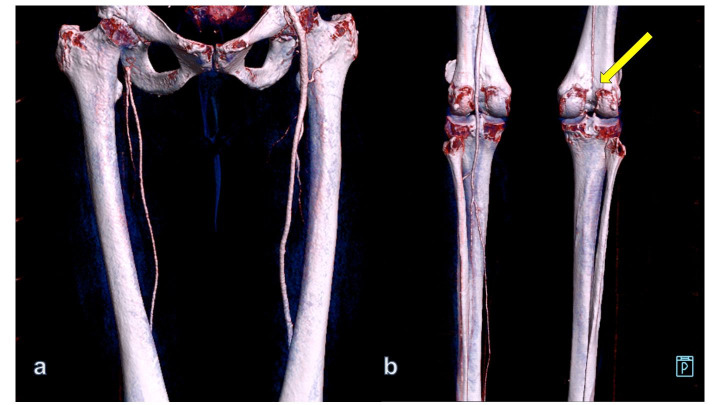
Angio CT with volume rendering 3D reconstruction showing infrafilling of a patent right superficial femoral artery (a). Total occlusion of the right popliteal artery (arrow) with absence of tibial vessels (posterior view). In the left lower extremity, all infrapopliteal vessels are patent (b), (patient #1).
